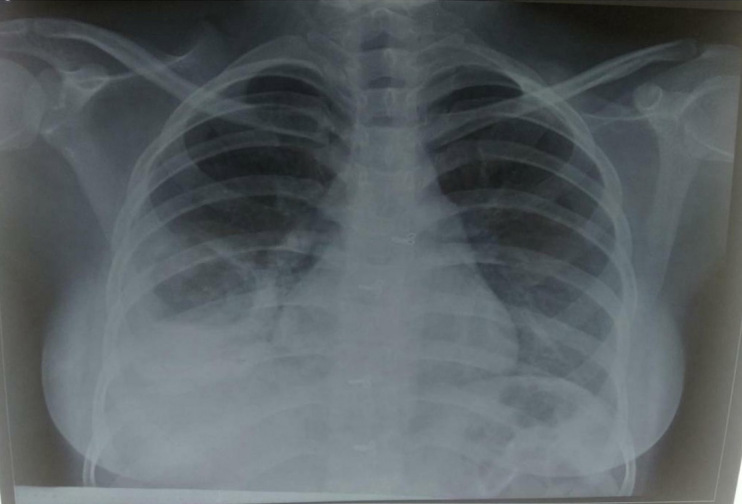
Chest X-Ray with abdominal shield suggestive of bilateral inhomogeneous infiltrates and a thick-walled cavity with air-fluid level in the lower zone of the right lung field.
